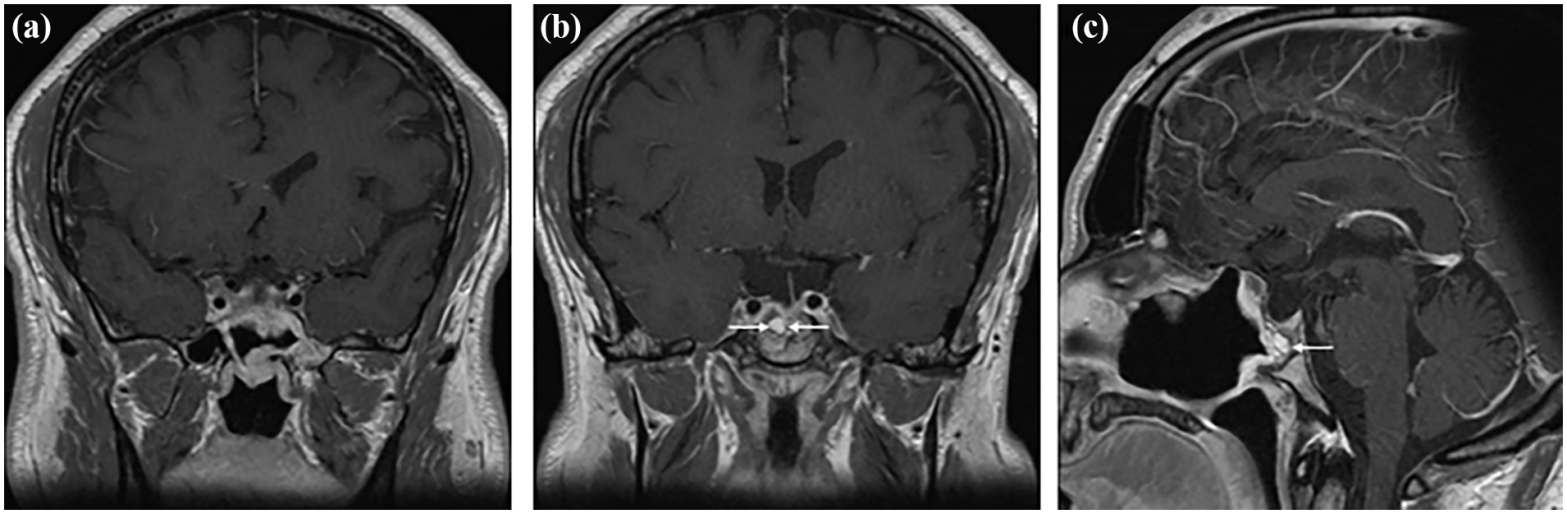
Postoperative MRI. Coronal (a, b) T1 post-gadolinium MRI showing resection of the tumor including the extension into the cavernous sinus. Sagittal reconstruction (c) demonstrating nasoseptal flap reconstruction of the sella.
